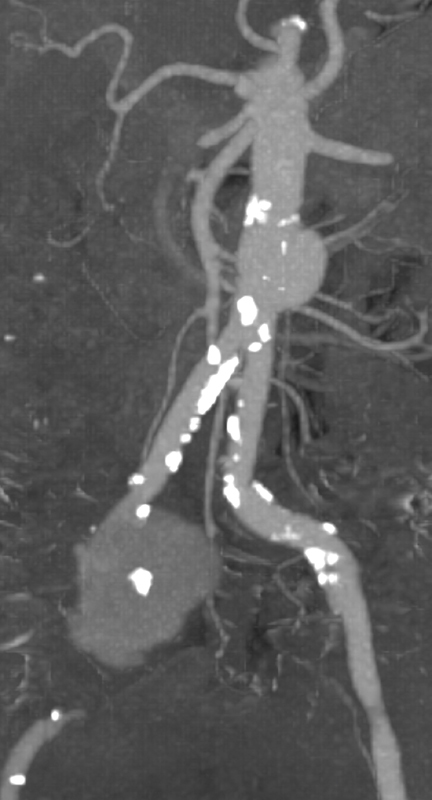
Maximum intensity projection of CTA showing both the isolated abdominal aortic and right common iliac artery aneurysm. CTA, computed tomography angiography.
